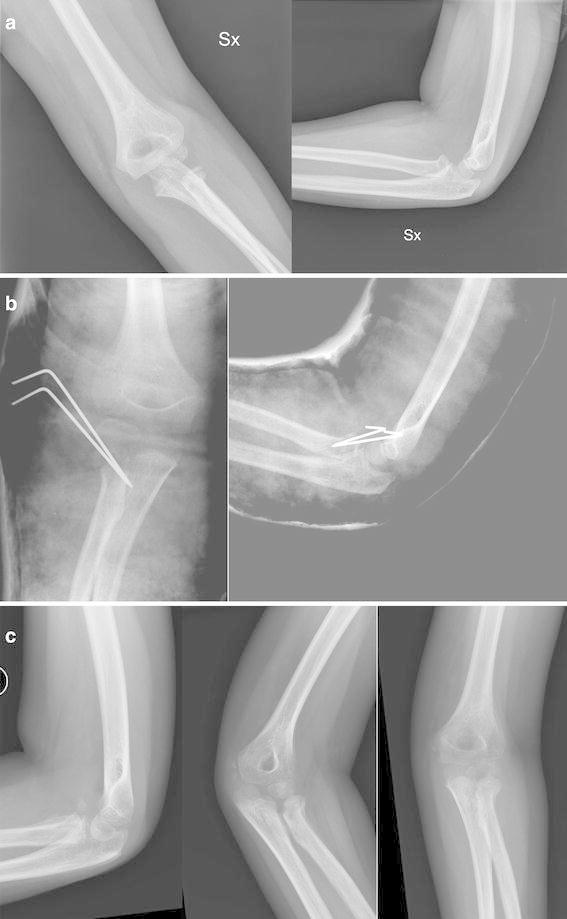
a Radial neck fracture Judet type III. b Osteosynthesis with two percutaneous K-wires, postoperative X-rays. c X-rays at 3 months, after K-wires removal, showing a good alignment
